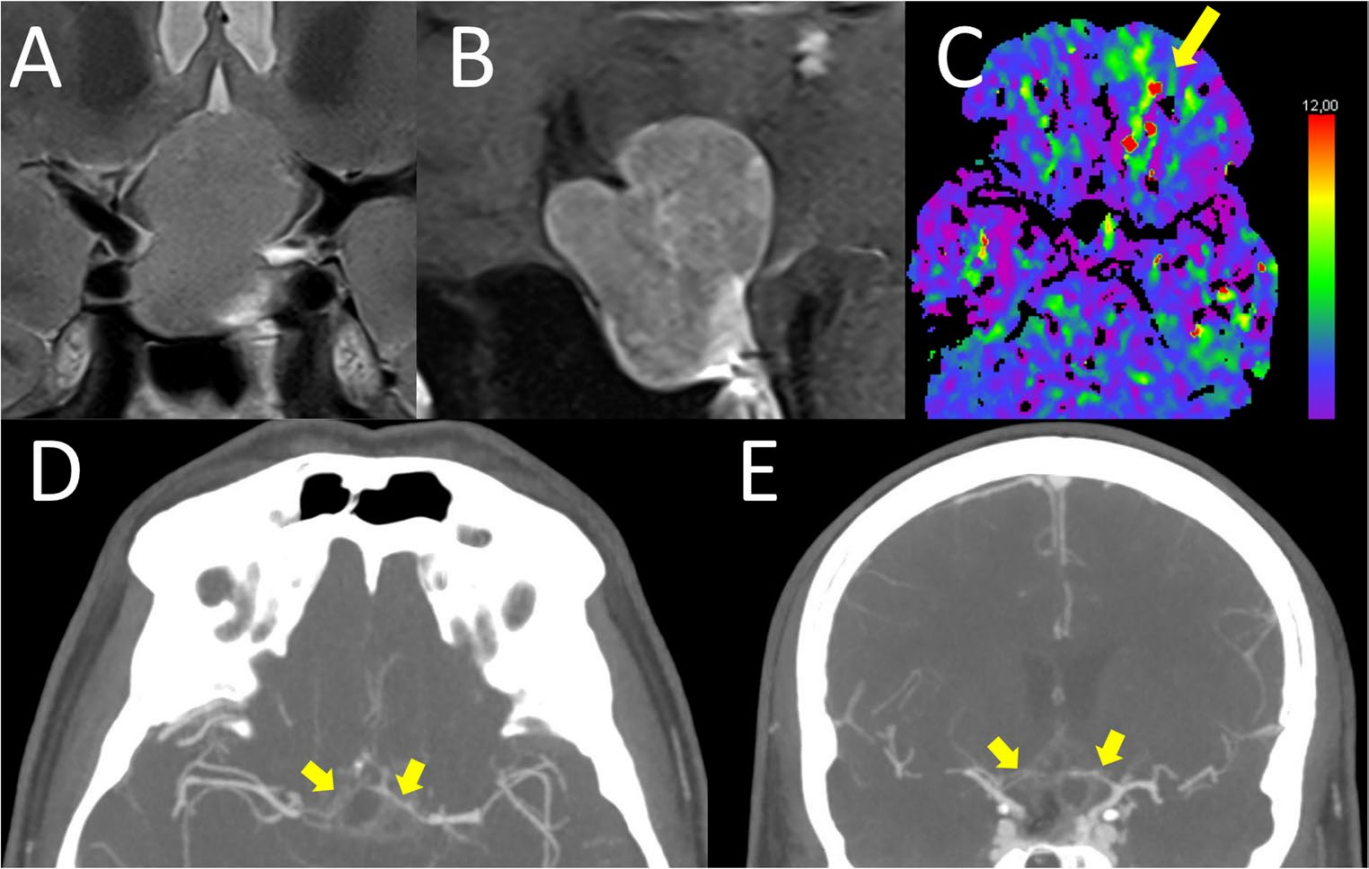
Case 1–51 year old male with lactotroph pituitary neuroendocrine tumor. A : preoperative T2-weighted MR coronal section showing a sellar tumor with suprasellar extension elevating the floor of the third ventricle. B: preoperative sagittal T1-weighted contrast-enhanced MR section demonstrating two suprasellar tumor extensions above the tuberculum sella towards the anterior cerebral arteries as well as towards the third ventricular floor. C: Postoperative day 12, Tmax map of perfusion CT, arrow pointing out spots of delayed perfusion in the left orbitofrontal area supplied by the anterior cerebral artery. D (axial) & E (coronal): contrast-enhanced CT angiography on postoperative day 12, arrows marking extreme vessel narrowing of A1 segment of both anterior cerebral arteries indicative of vasospasm
